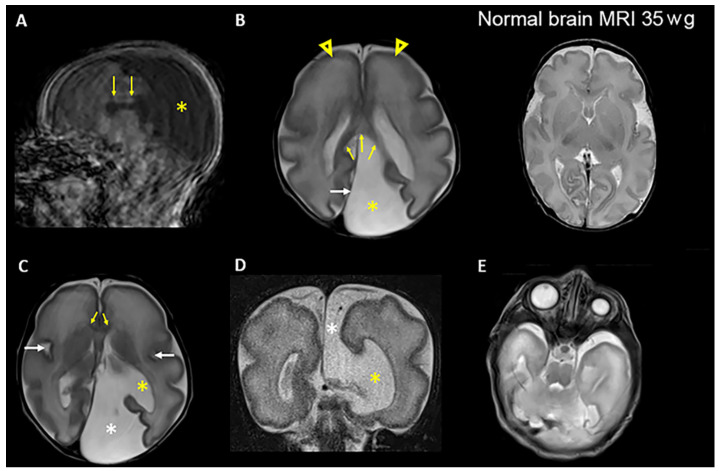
Brain MRI images of patient 1 in the postnatal period at approximately 35 weeks gestational age demonstrate multiple congenital anomalies. (A) Sagittal T1-weighted image shows the presence of the corpus callosum, which is not properly developed (arrows). The posterior portion (the splenuim) is located further anterior than normal. CSF fills most of the posterior supratentorial compartment (asterisk). Note motion artifacts in the basal regions of the brain. (B) Left: Axial T2-weighted image shows an expansion of the left supratentorial cranial cavity (interhemispheric cyst) filled with CSF (asterisk) in the parietooccipital region of the left hemisphere. The posterior falx cerebri (white arrow) is shifted toward the right hemisphere. The splenium of the corpus callosum is visible (yellow arrows). There is primitive gyrosulcal patterning, more prominent in the frontal lobes (arrowheads), consistent with 28–29 weeks of gestation. Right: Axial T2-weighted image shows normal gyrus and sulcus formation at 35 weeks of gestation (wg). (C) Axial T2-weighted image shows the interhemispheric cyst (white asterisk) communicating with the enlarged left lateral ventricle (yellow asterisk). The genu of the corpus callosum is visible (yellow arrows). The sylvian fissures (white arrows) appear underdeveloped in both hemispheres. (D) Coronal T2- weighted image shows the communication of the CSF space (interhemispheric cyst) (white asterisk) with the lateral ventricle (yellow asterisk) on the left side through a broad parenchymal defect in the medial temporoparietal region. (E) Axial T2-weighted image shows pronounced left microphthalmia, without detectable lens.
